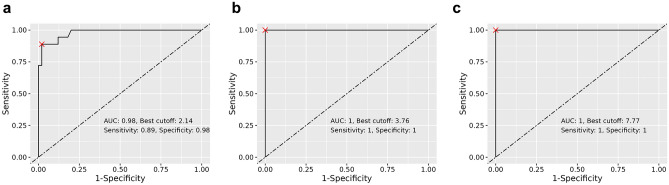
ROC analysis of SUVmax for differentiating the visual grade, DS. SUVmax values of 2.14, 3.76 and 7.77 were selected as the optimal cutoff values for differentiating DS 0/1 (a), 1/2 (b), and 2/3 (c), respectively.
